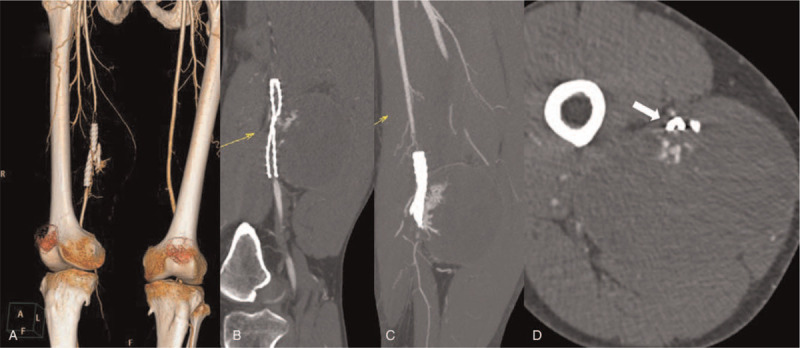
Computed tomography angiography when readmitted. (A) The stent–graft was occluded, and a material of high density was adjacent to the stent–graft. (B) The stent–graft was compressed by the pseudoaneurysm. (C) The pseudoaneurysm was derived from the side-branch of the deep femoral artery. (D) The stent–graft was fractured and occluded (white arrow).
